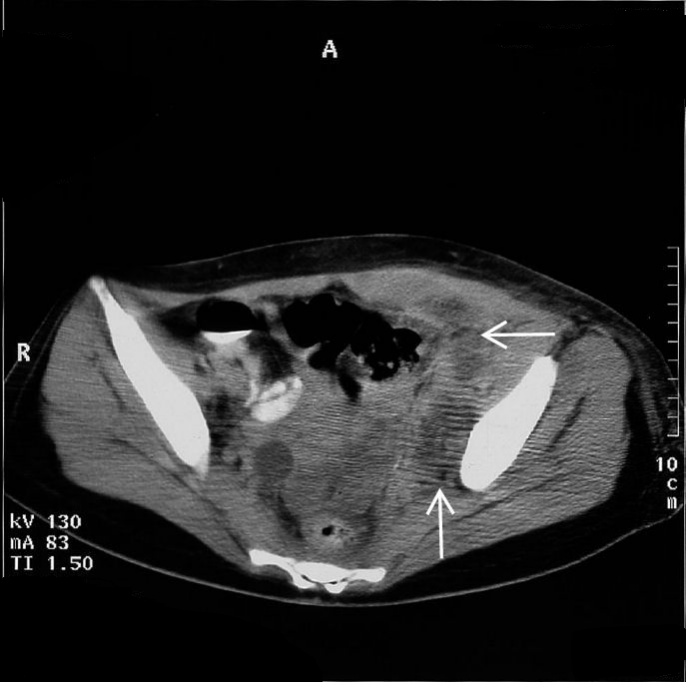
Computed tomographic scan of the abdomen showing multiple small abscesses in the left iliopsoas (arrows) that established the diagnosis of pyomyositis.
